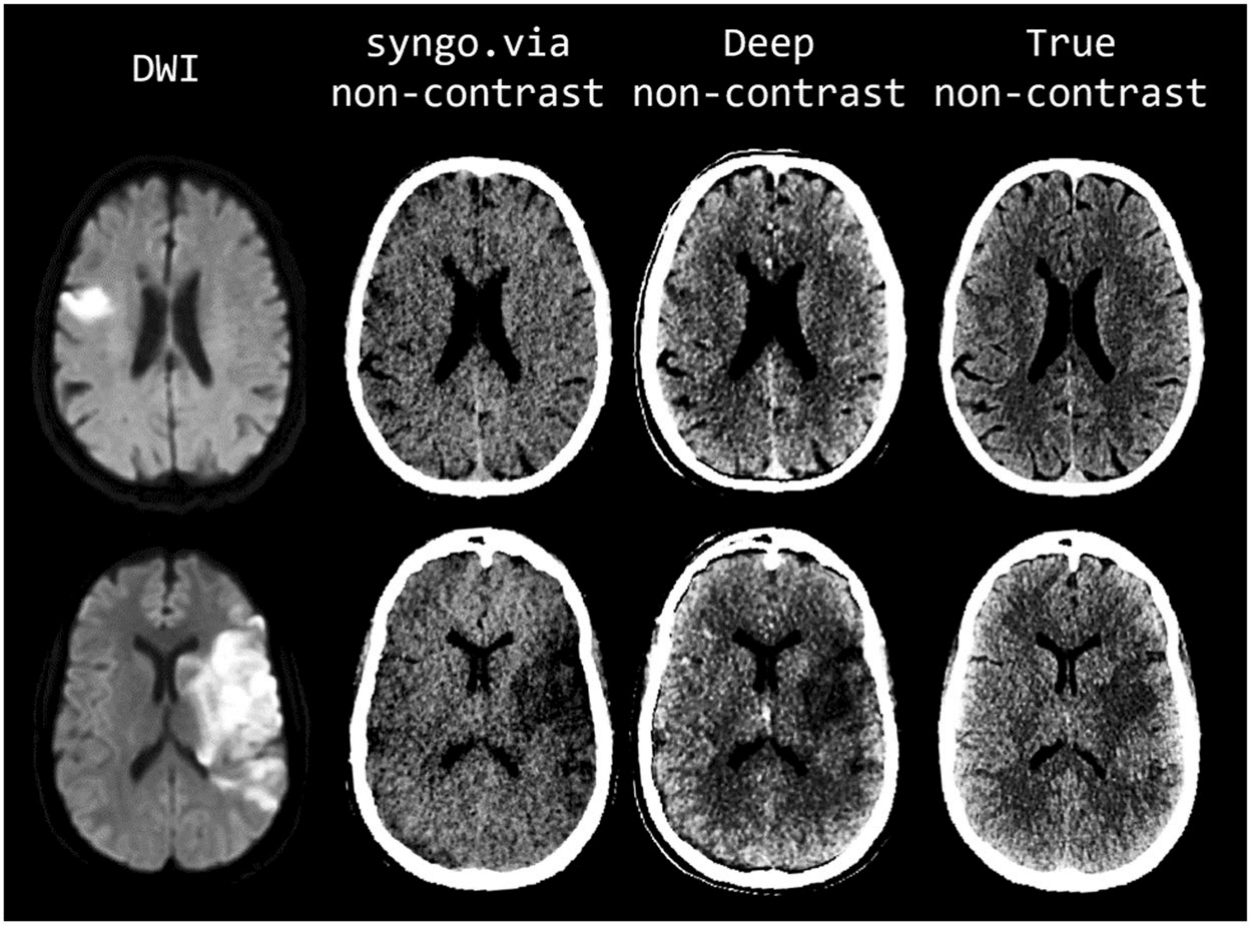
Comparison of representative examples of diffusion-weighted images (first column), S-VNC images reconstructed using a conventional material decomposition algorithm (second column, display window of (20, 60)), predicted DNC images generated using the proposed CNN (third column, display window (40, 80)), and TNC images (fourth column, display window (40, 80)) in the evaluation of acute ischemic stroke. Decreased SNR on the VNC images results in poor delineation of true infarct size and location. In contrast, the improved image quality of the predicted DNC images allows for better visualization of normal brain anatomy and pathology, as confirmed by DWI. First row: 75-year-old male with acute-onset left facial droop and left arm weakness secondary to an acute ischemic infarct within the anterior right middle cerebral artery territory. Loss of gray-white matter differentiation and local sulcal effacement secondary to the infarct are best visualized on the predicted DNC image in comparison to the VNC and TNC images. Of note, identification of the infarct is particularly difficult on the VNC image. Second row: 51-year-old unresponsive female who presented with a large acute left middle cerebral artery territory infarction. The true size and location of the acute infarct, as confirmed by DWI imaging, is most conspicuously identified on the predicted DNC image secondary to improved SNR.
